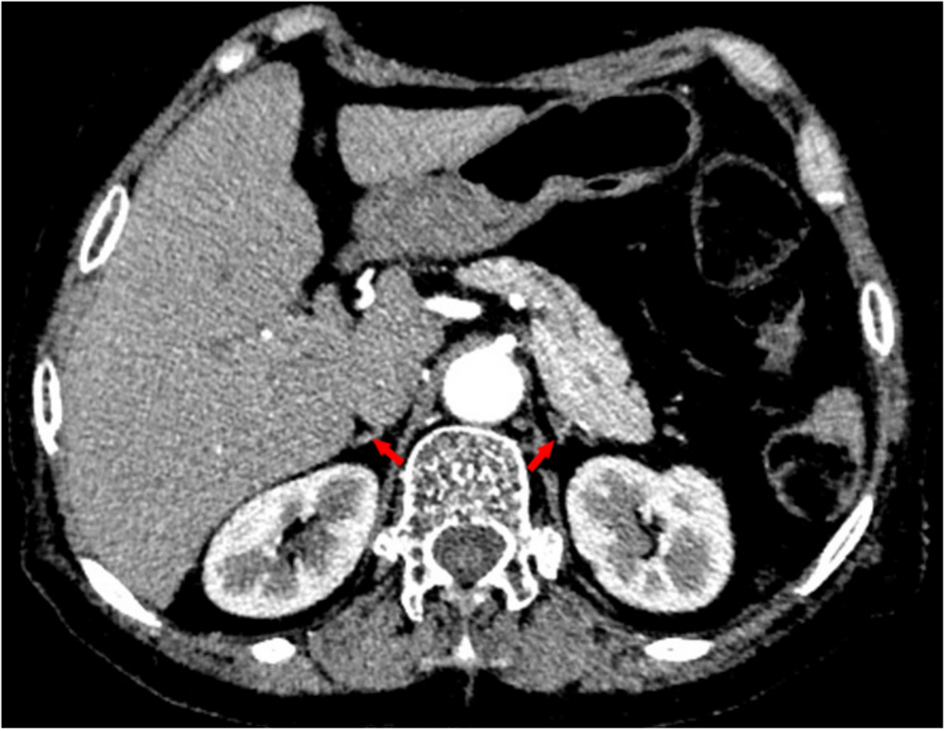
Contrast-enhanced computed tomography revealing atrophic bilateral adrenal glands (red arrows).
